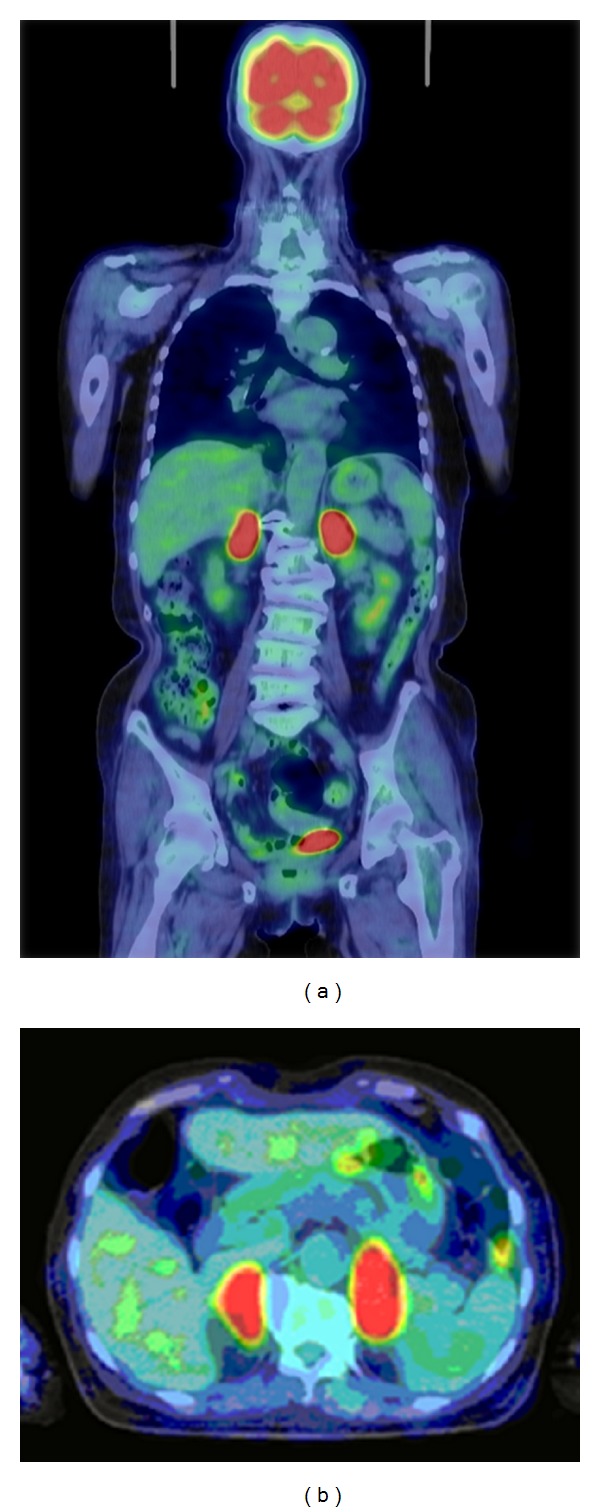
FDG-PET image showing an exclusive strong uptake in both adrenal glands: (a) coronal section; (b) horizontal section.
